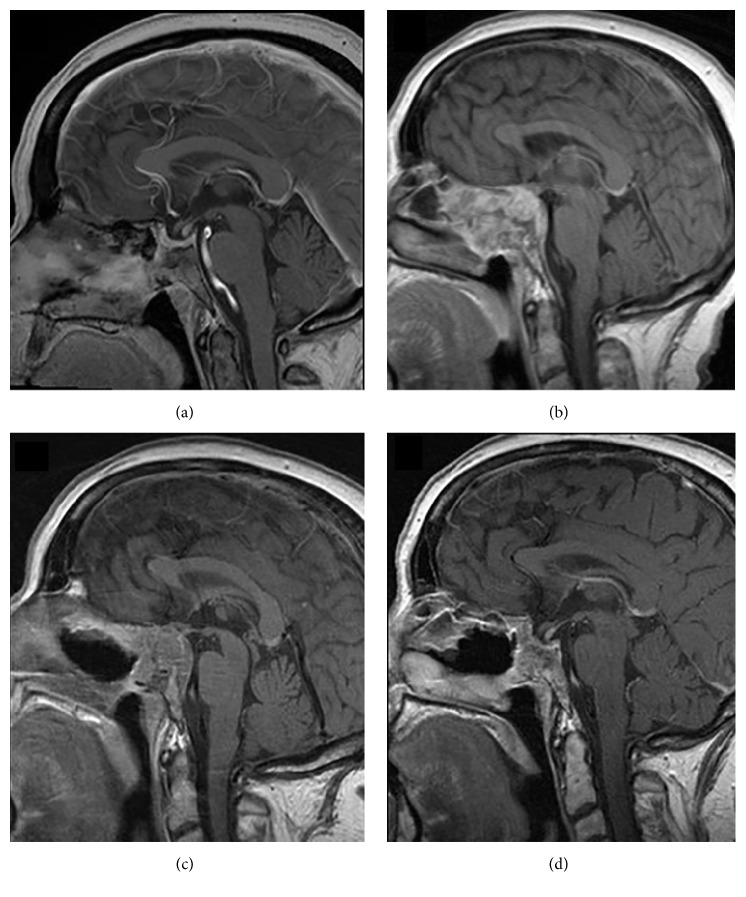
Pituitary MRI appearances (T1 postgadolinium weighted sagittal images). (a) After the first transsphenoidal operation for macrocorticotropinoma in 2010 with a small tumor remnant. (b) Three years after the first operation. Pituitary adenoma with destruction of the floor of the sella and invasion into sphenoid sinus and both cavernous sinuses. (c) Three months after the initiation of the concurrent therapy with TMZ and radiotherapy. (d) After the 6 cycles of TMZ. Stable pituitary remnant and biochemical control of the disease.
